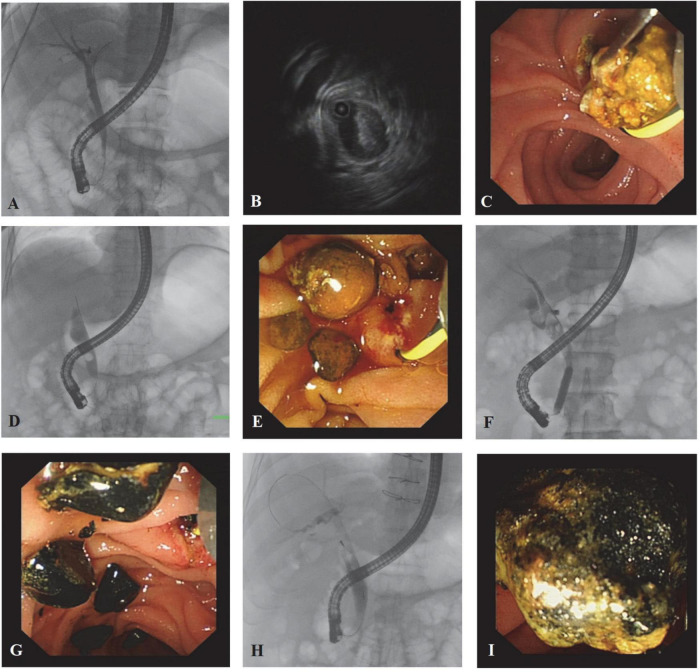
Cholangiographic, IDUS, and endoscopic images from typical cases. Patient 1: A 37-year-old male choledocholithiasis patient was diagnosed with abdominal ultrasonography. (A) The cholangiographic image failed to reveal the stones. (B) IDUS confirmed the presence of stones in the bile duct, but the number of stones was not accurate. (C) During lithotomy, a large amount of sludge was pulled out. Since more than three extractions were done, the score of stones was modified as score 3. Patient 2: A 65-year-old female patient diagnosed with choledocholithiasis by abdominal CT scan. (D) Only one stone was found under the shaft of the endoscope, while stones inside the end of the common bile duct could not be seen. (E) More than five brownstones were extracted, and most of them existed in the lower part of the common bile duct. Patient 3: A 75-year-old male choledocholithiasis patient was diagnosed with abdominal ultrasonography. (F) Cholangiography demonstrated many stones in the middle part of the common bile duct, and stones in the cystic duct can also be seen. (G) Black calculus with different sizes was taken out. Patient 4: A 68-year-old male patient was diagnosed with choledocholithiasis by abdominal CT scan. (H) Stones <1 cm were shown above the shaft by cholangiography. (I) The long diameter of the stone was around 1.2 cm.
